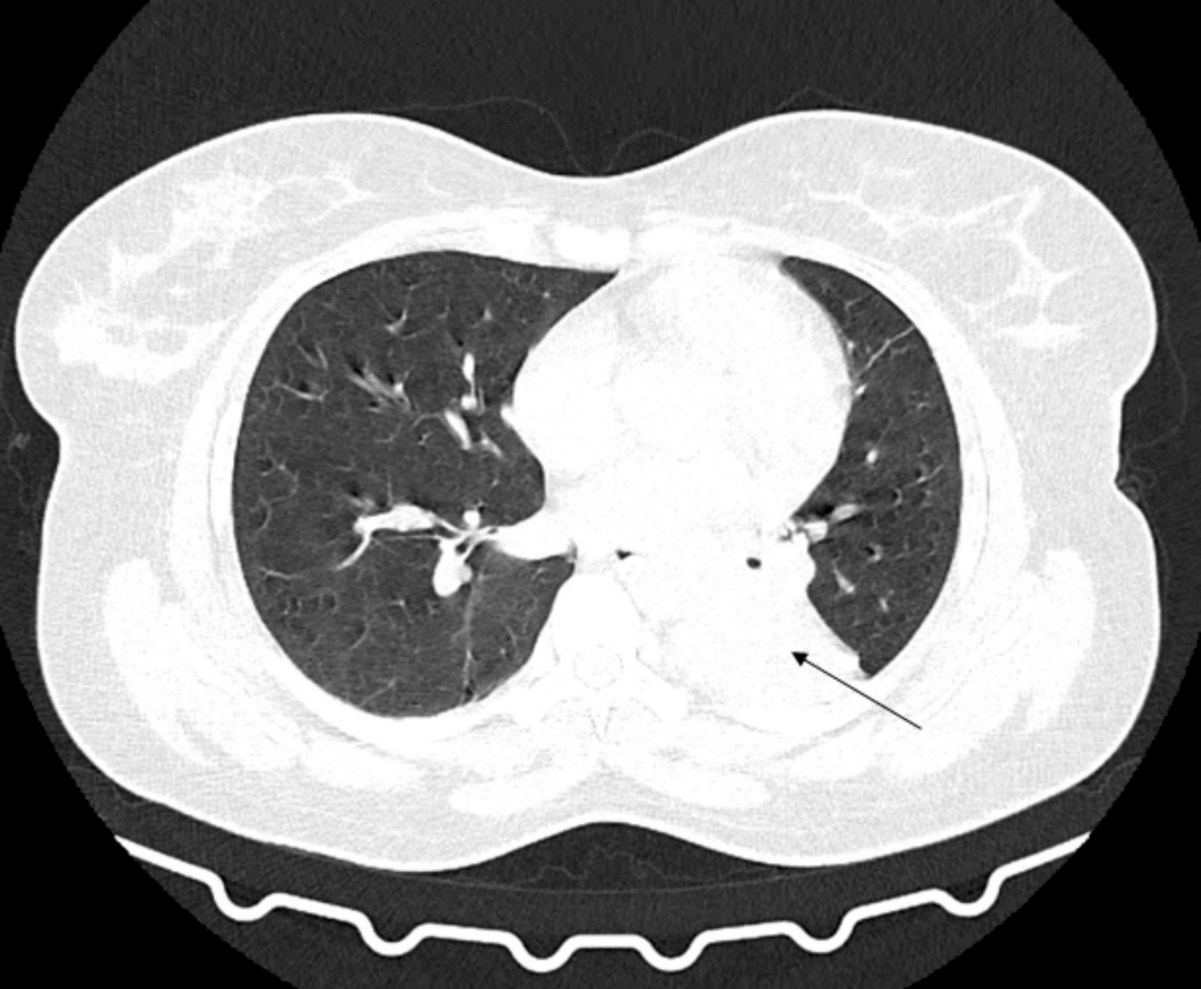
Computed tomography (CT) axial section of the thorax, using the lung window, reveals a soft tissue lesion in the left lower lobe, as indicated by the black arrow.
